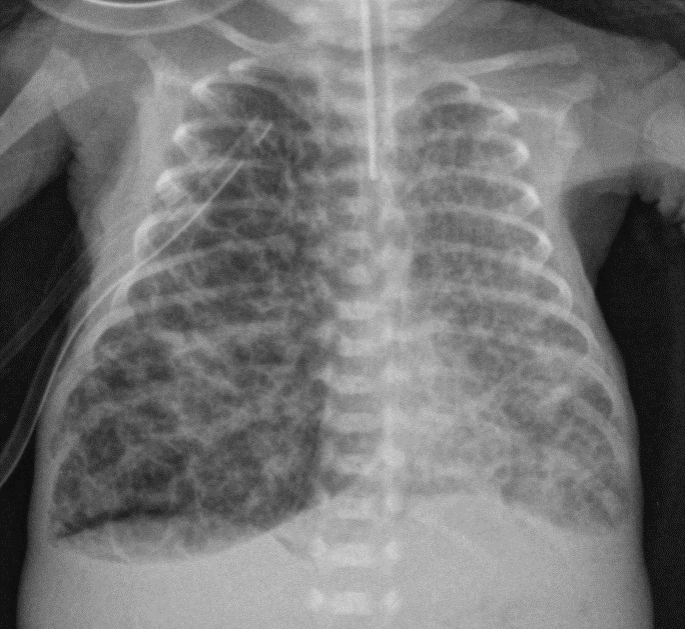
Supine chest radiograph at 25 days of age with chest tubes placement shows persistent extensive bubbly lucencies in both lung (PIE) and resolution of the most of right pneumothorax (slight shift of the heart leftward pressing the left lung and the depression of the right hemidiaphragm is still visible).
